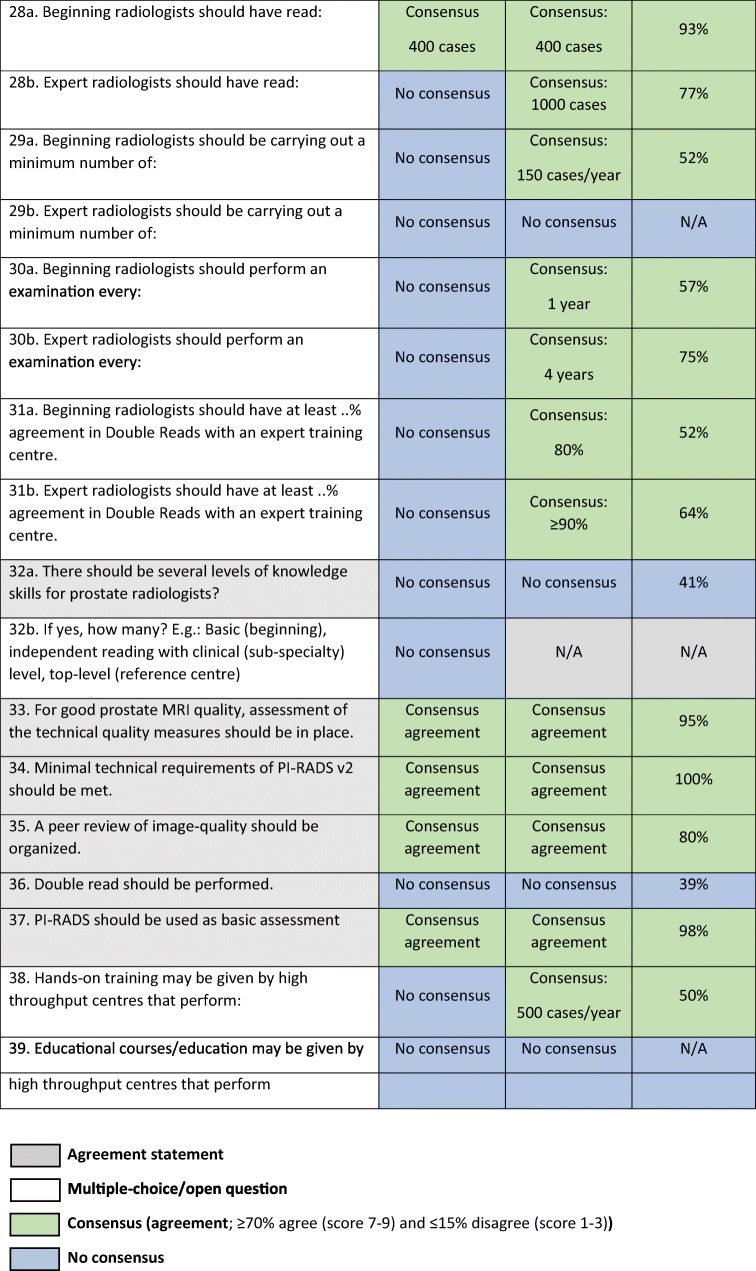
ESUR/ESUI consensus outcomes for section 3: Experience and training centres. *ESUI* EAU Section of Urologic Imaging, *ESUR* European Society of Urogenital Radiology, *MDT* multidisciplinary team, *mpMRI* multi-parametric MRI, *N/A* not applicable
